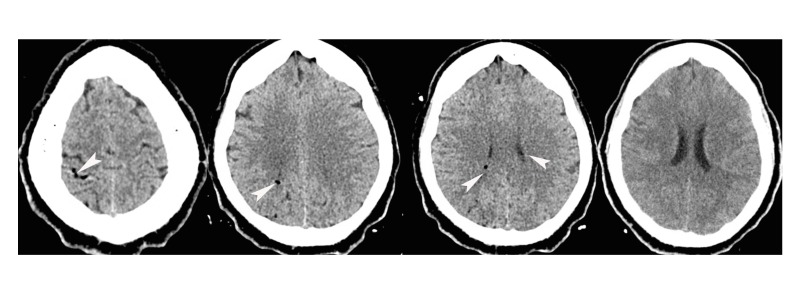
Axial non-contrast computed tomography (CT) of the head. Head CT with arrows point to intravascular pockets of air on several cuts.
